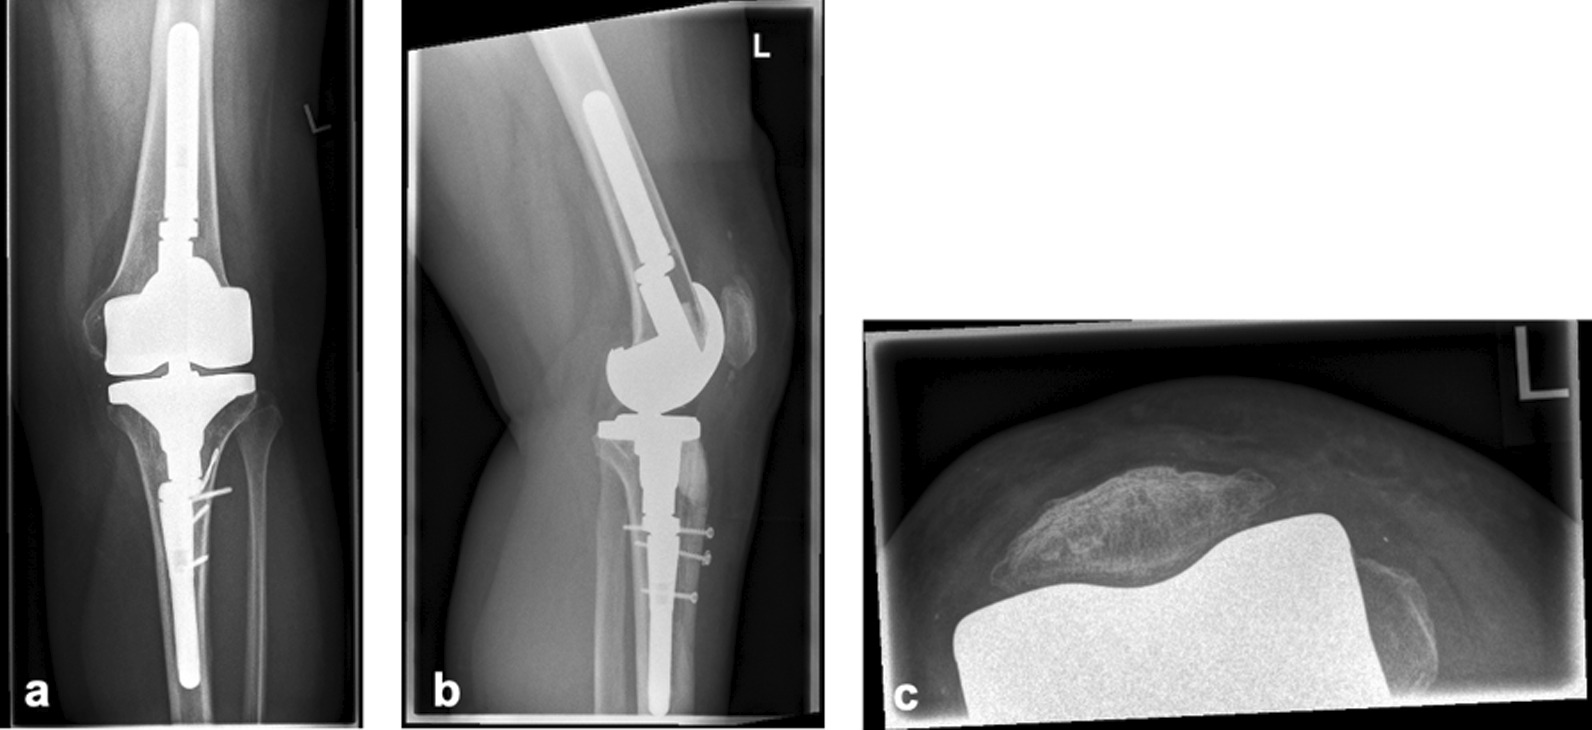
Radiologic follow up after ten month: X-ray shows correct implant position without periprosthetic bone lesions and centered patella tracking. a Frontal view. b Sagittal view shows still correct position of cement augmentation. c Axial view shows regular patella tracking
